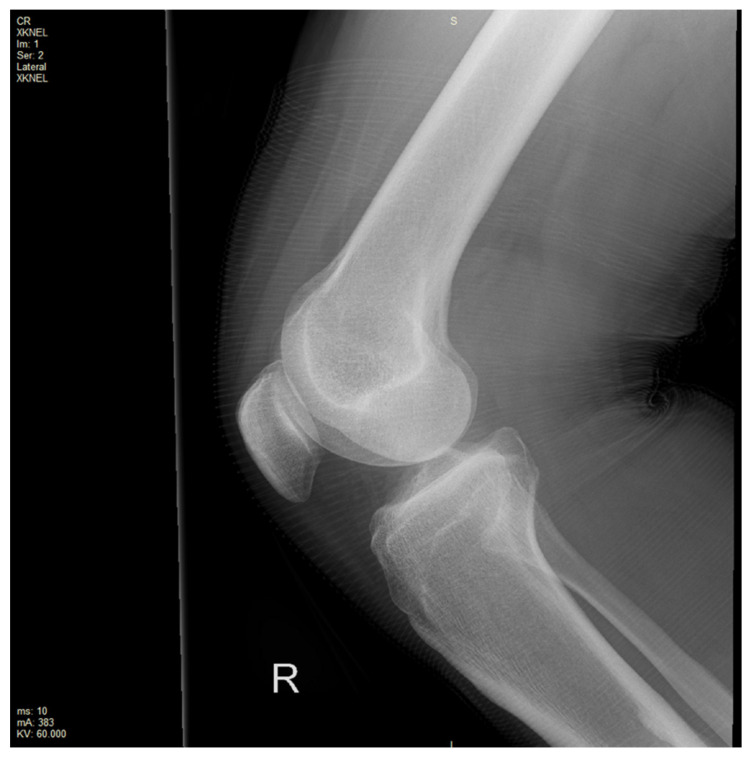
X-ray of the right knee with a soft tissue shadow extending into the supra-patellar recess.
